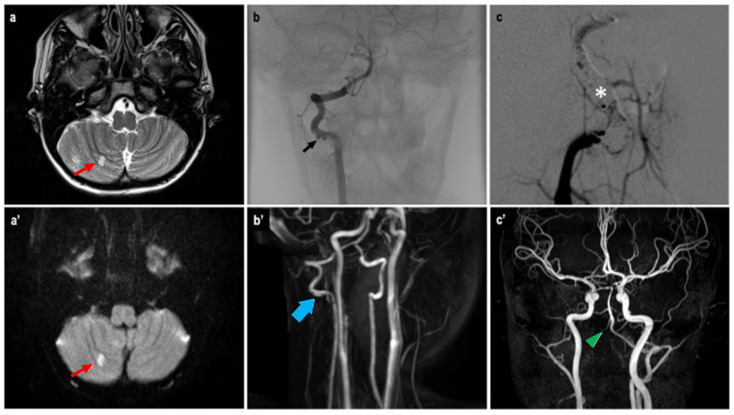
Angio-MRI T2w (a) and DWI (a’) sequences showing a hyperintense area (red arrow) of the right cerebellar hemisphere, a possible ischemic consequence of the suspected vasospasm of the posterior cerebral circulation. Angiographic study (b) confirmed a floating thrombus at the right VA previously described as a VA stenosis at the Angio-MRI (b’). A flow-diverter placement was not possible, thus requiring a coiling (white asterisk) of the VA sparing the PICA (c). The control Angio-MRI (c’) showed the exclusion of the right VA after the endovascular procedure (green arrowhead).
